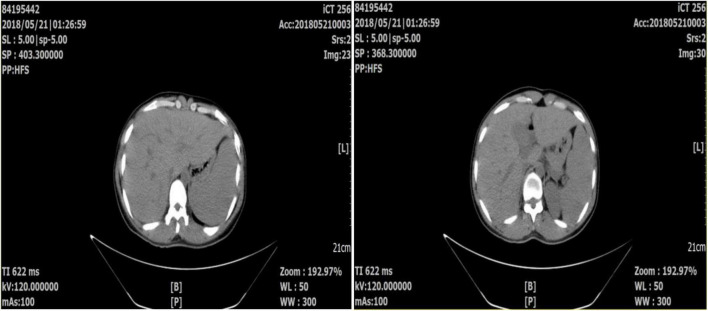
Abdominal computed tomography showed no splenic infarction.
